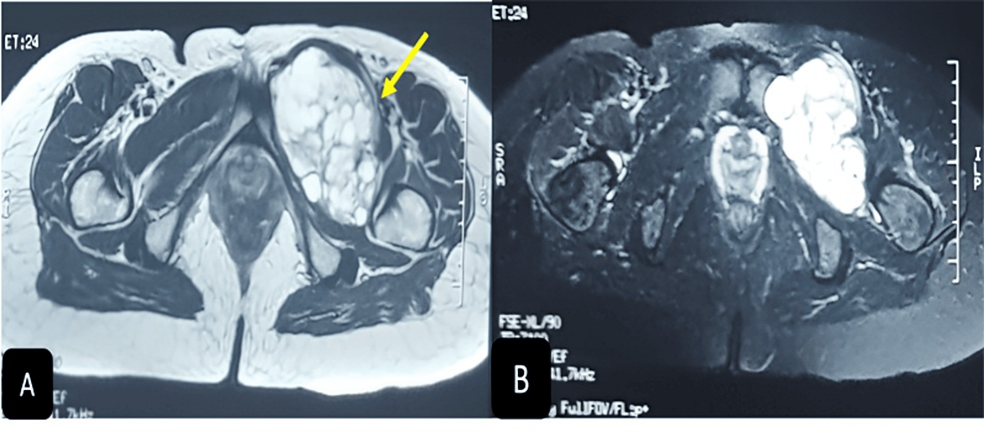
Axial section of the MRI; T1 (A) and T2 (B) signals are visualized
